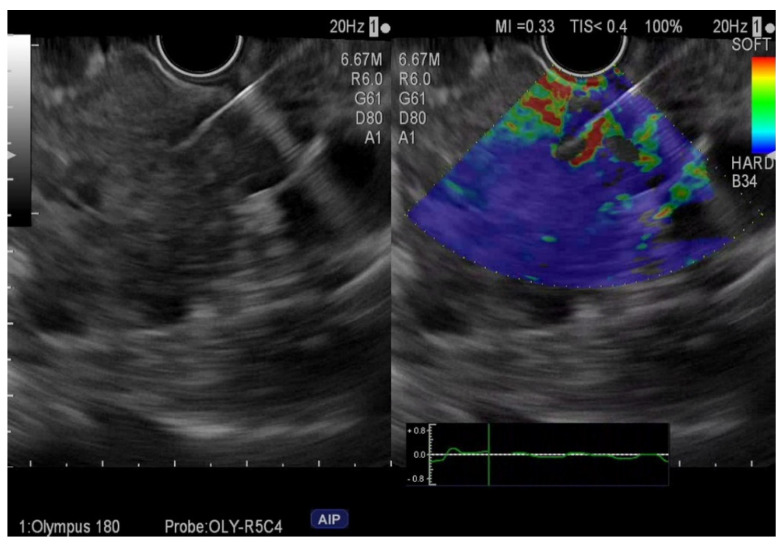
Elastography endoscopic ultrasound fine-needle aspiration of pancreatic adenocarcinoma.
